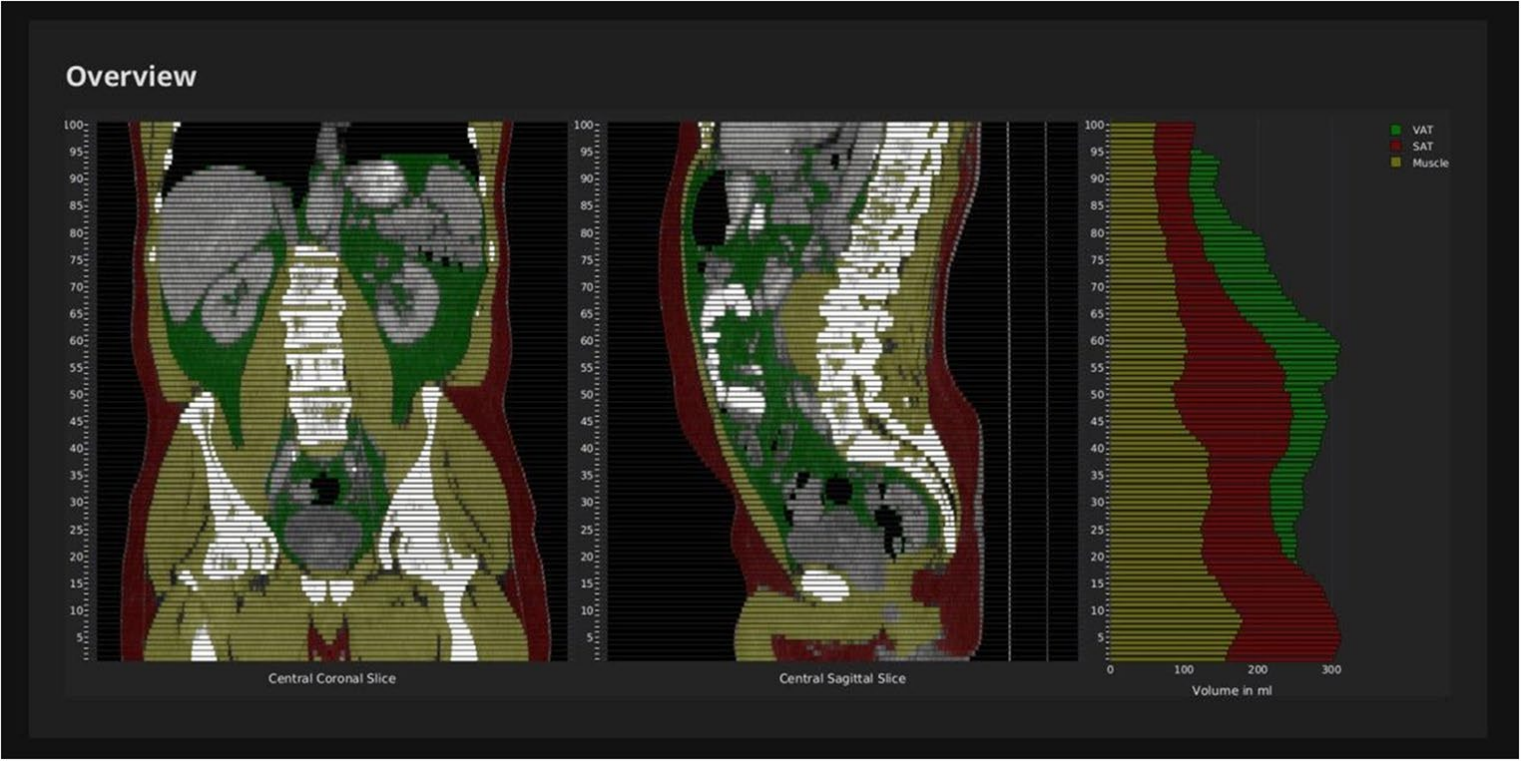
CT-Abdomen for the assessment of the total volume of subcutaneous- and visceral adipose tissue and muscle tissue using convolutional neuronal network
